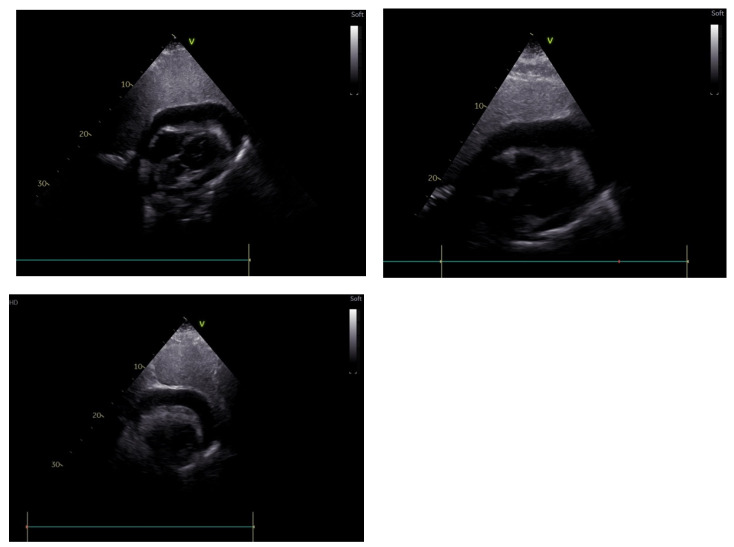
Transthoracic echocardiogram showing a large pericardial effusion (most prominent around the left ventricular posterior wall and the left ventricular apex with about 2.7 cm and 2.4 cm in diameter, respectively) with a ventricular systolic collapse and a ‘’swinging heart’’ view.
